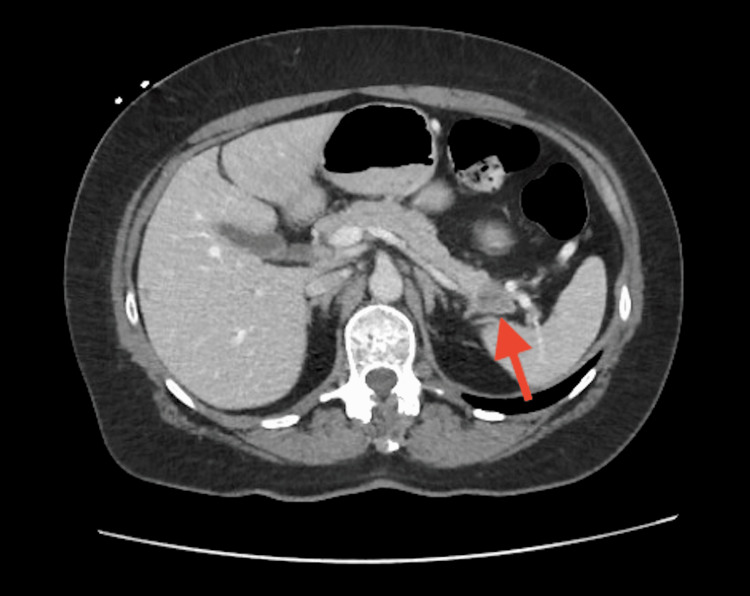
Pancreatic malignancy on CT of the abdomen with contrast. Malignancy screening with CT of the chest, abdomen, and pelvis was significant for a distal pancreatic body/tail mass (red arrow) concerning for a primary malignancy and multiple liver lesions concerning for metastasis.
